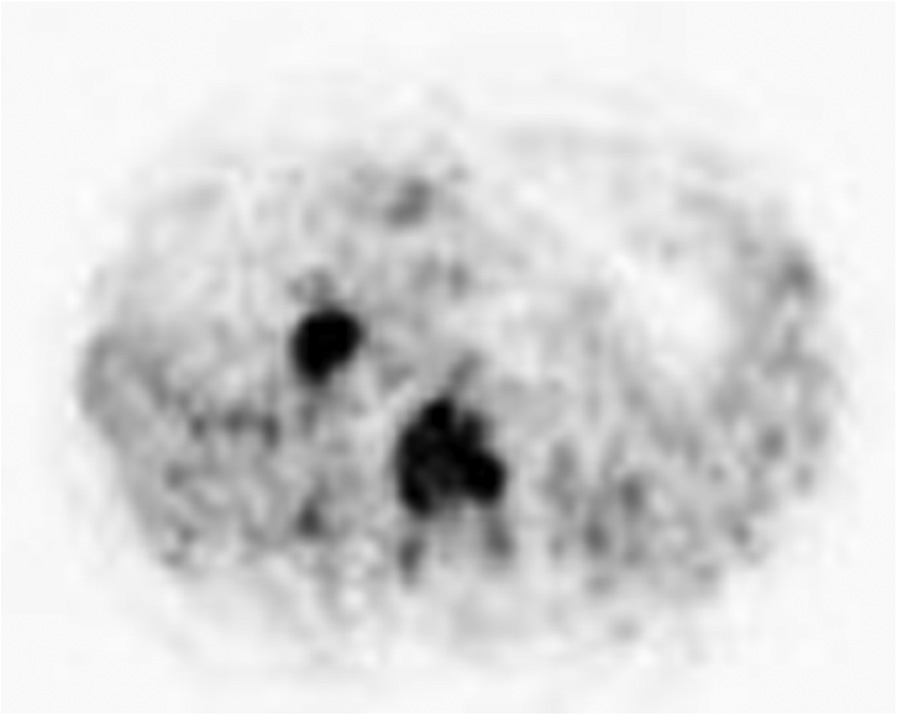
In axial PET image, FDG 18 (fluorodeoxy-glucose) uptake is seen in the periampullary region
